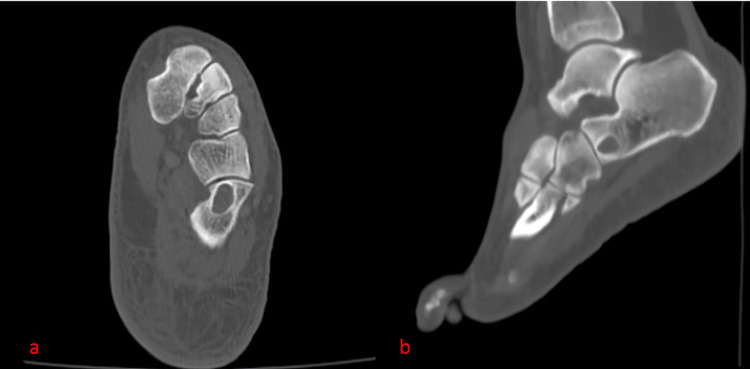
Mass surrounded by sclerotic rim adjacent to the calcaneocuboid joint in CT axial (a) and sagittal (b) images
